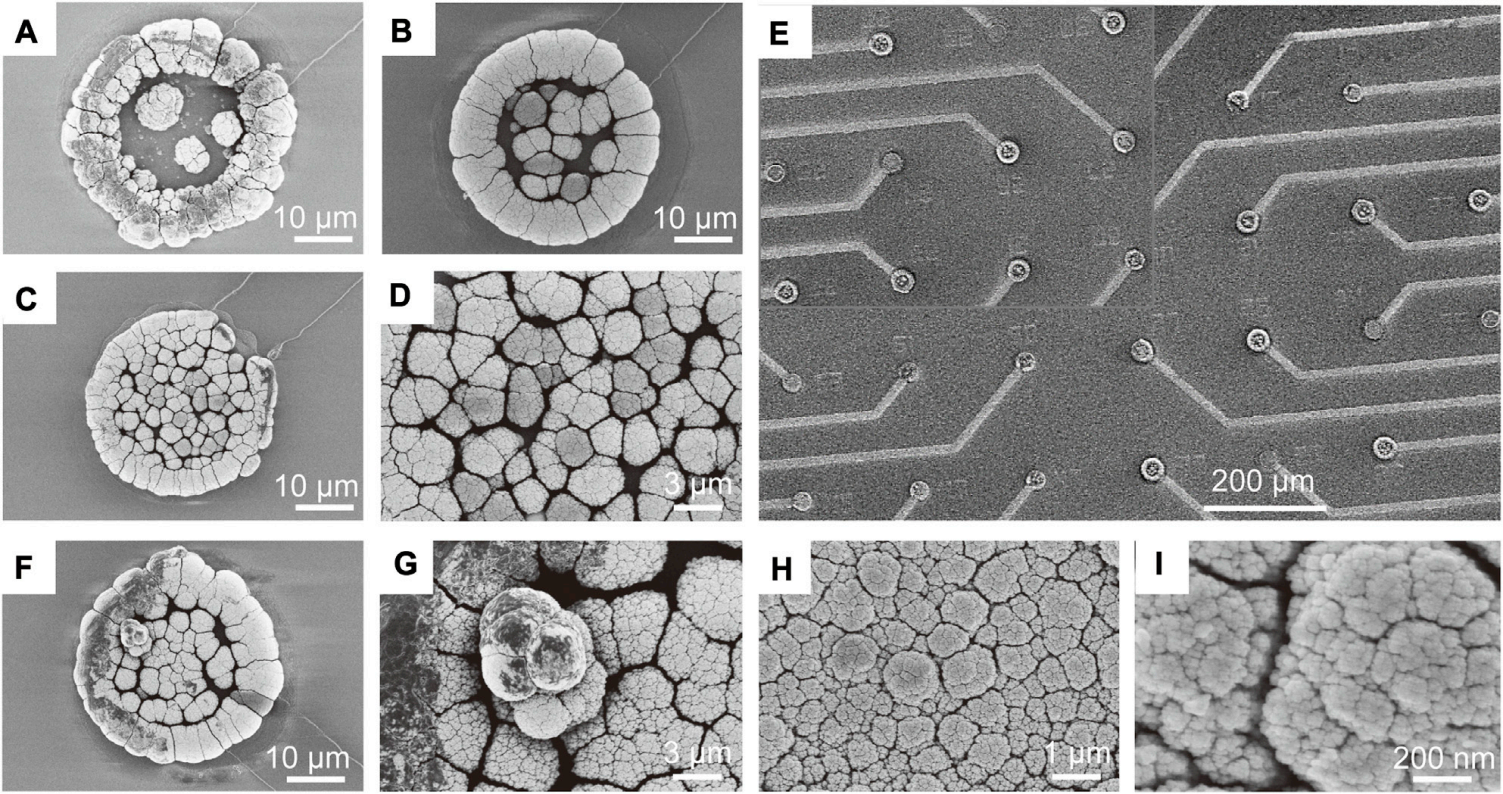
Morphological characterization of 3D MEA. (A) Morphology of PtNPs growing adherently to the wall to form a 3D ring structure. (B) Surface morphology of PtNPs deposition on the electrode without ultrasound. (C) Surface morphology of PtNPs deposition on the electrode with ultrasound. (D) SEM image of Figure 3C magnified to observe the formation of a more compact structure of PtNPs on the electrode with ultrasound. (E) SEM image of multiple electrode sites with protruding PtNPs formed by modification. (F) PtNPs forming a three-dimensional structure through layer-by-layer stacking. (G) Further magnification of the stacking details in Panel (F) reveals the clustering of multiple PtNPs, forming the three-dimensional structure. (H) Surface morphology of the electrode obtained with ultrasound in Panel (G), showing a more uniform distribution of PtNPs. (I) Morphology at the nanoscale in Panel (H), revealing a significant number of honeycomb-like structures.
